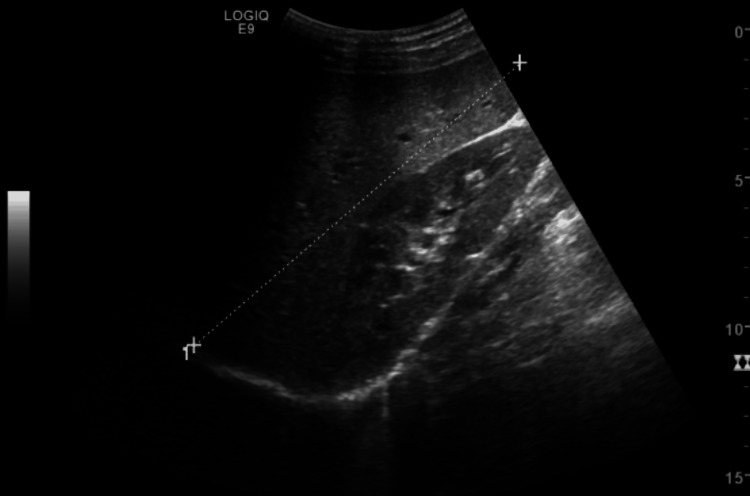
Abdominal ultrasound revealed moderate splenomegaly (154.8mm), with homogeneous parenchyma.
